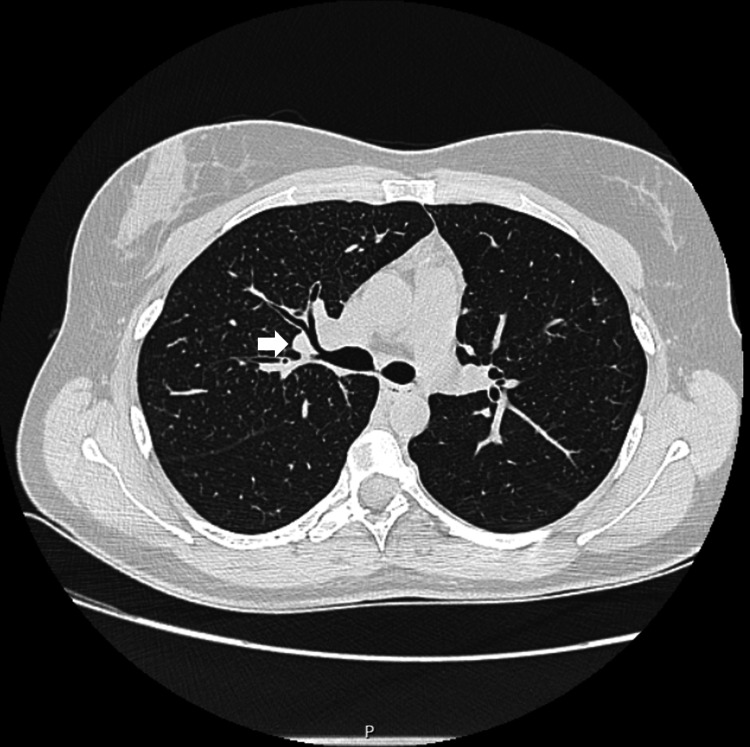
Third chest computed tomography scan showing the progression of the hilar lymphadenopathy clusters marked with an arrow (October 17, 2024).
